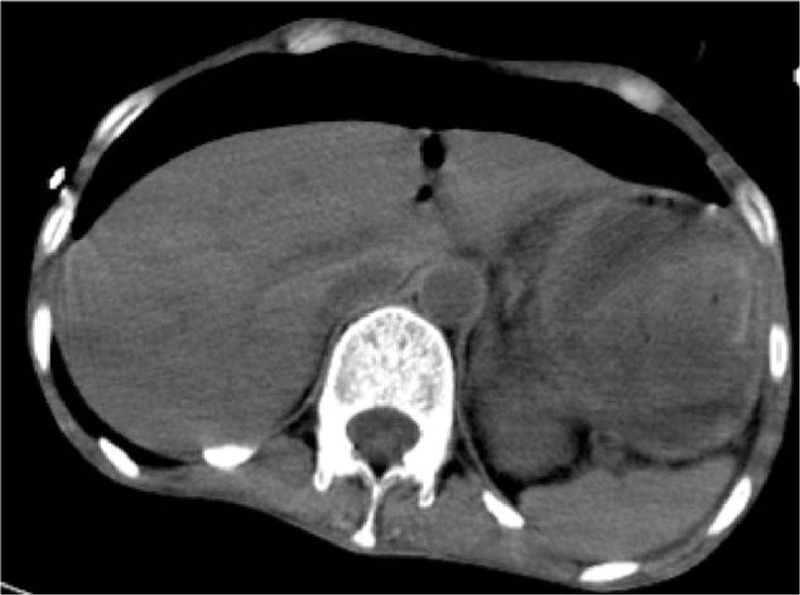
Enhanced computed tomography (CT) imaging of the abdomen. The patient underwent abdominal CT scanning at the time of hospitalization, and a large amount of gas within the peritoneal cavity was detected, which is known as pneumoperitoneum (PM).
